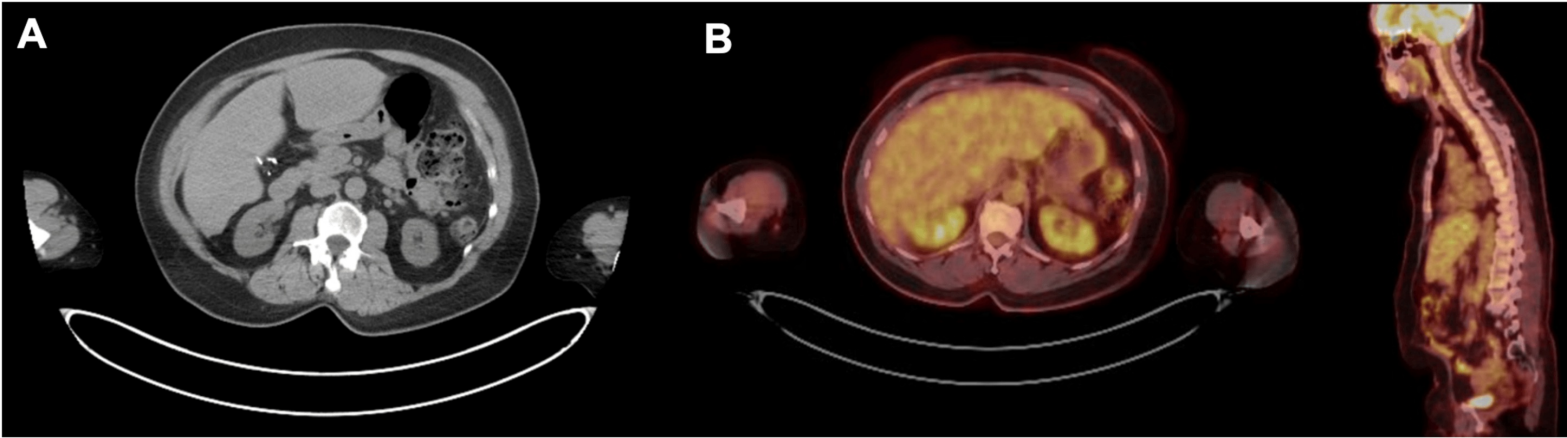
Routine surveillance imaging from January 2024 demonstrating no gastric involvement (A) Axial non-contrast CT of the abdomen showing no focal gastric wall thickening or mass. (B) Axial PET/CT of the abdomen demonstrating no hypermetabolic gastric activity. All images are from the same imaging time point and show no evidence of gastric metastasis.
